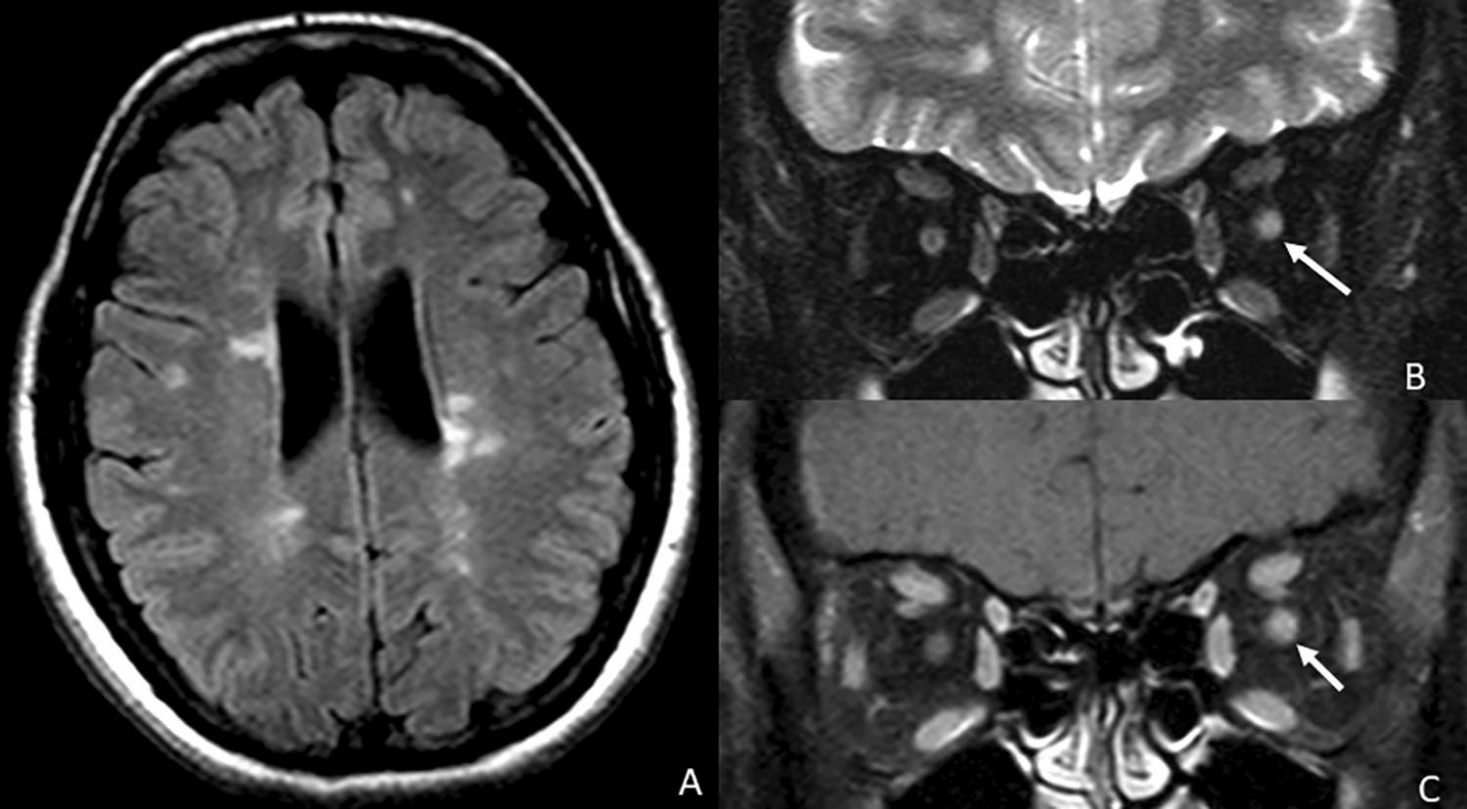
A case of a patient affected by multiple sclerosis with acute optic neuritis. A Axial FLAIR-weighted image showing multiple sclerosis-related hyperintense lesions in the periventricular white matter. B Coronal STIR-weighted image showing oedematous appearance of the left optic nerve in its intraorbital retrobulbar segment (arrow). That shows linear enhancement after contrast medium administration in c coronal T1 FS post-contrastographic weighted image (arrow)
